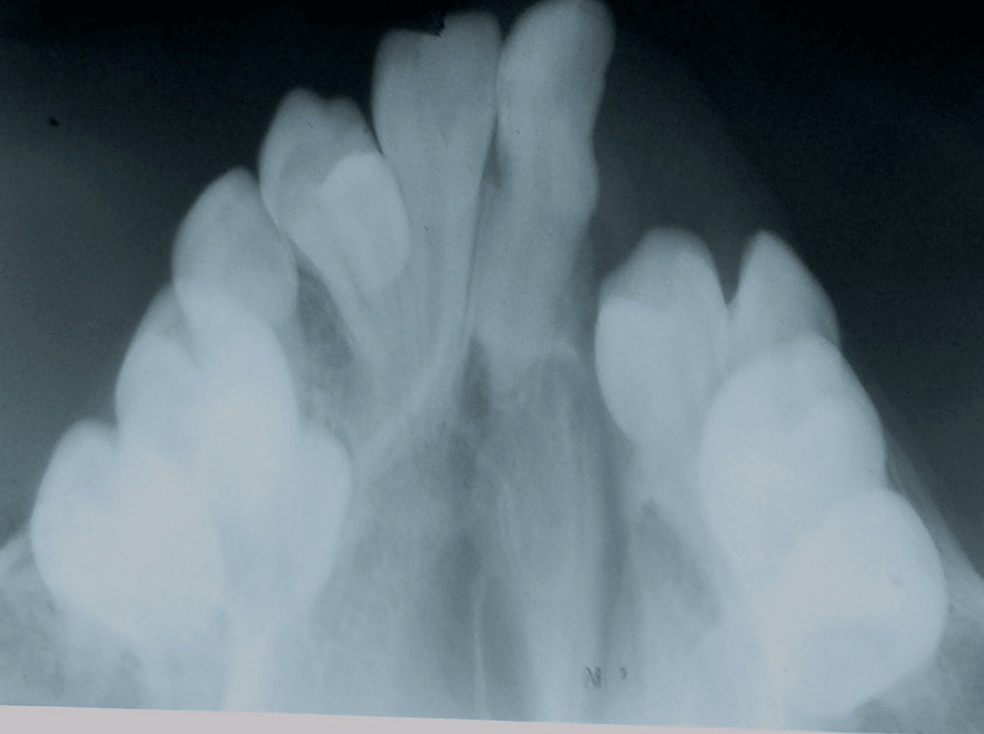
Occlusal radiograph showing cleft region in the alveolus
